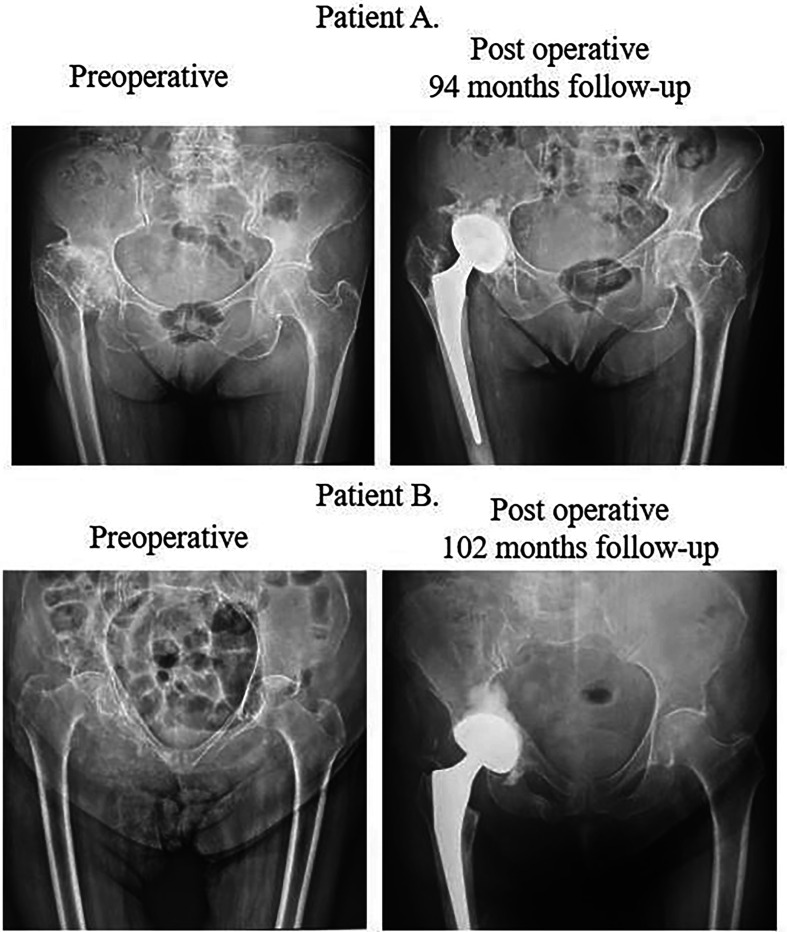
Radiographs of two patients.
